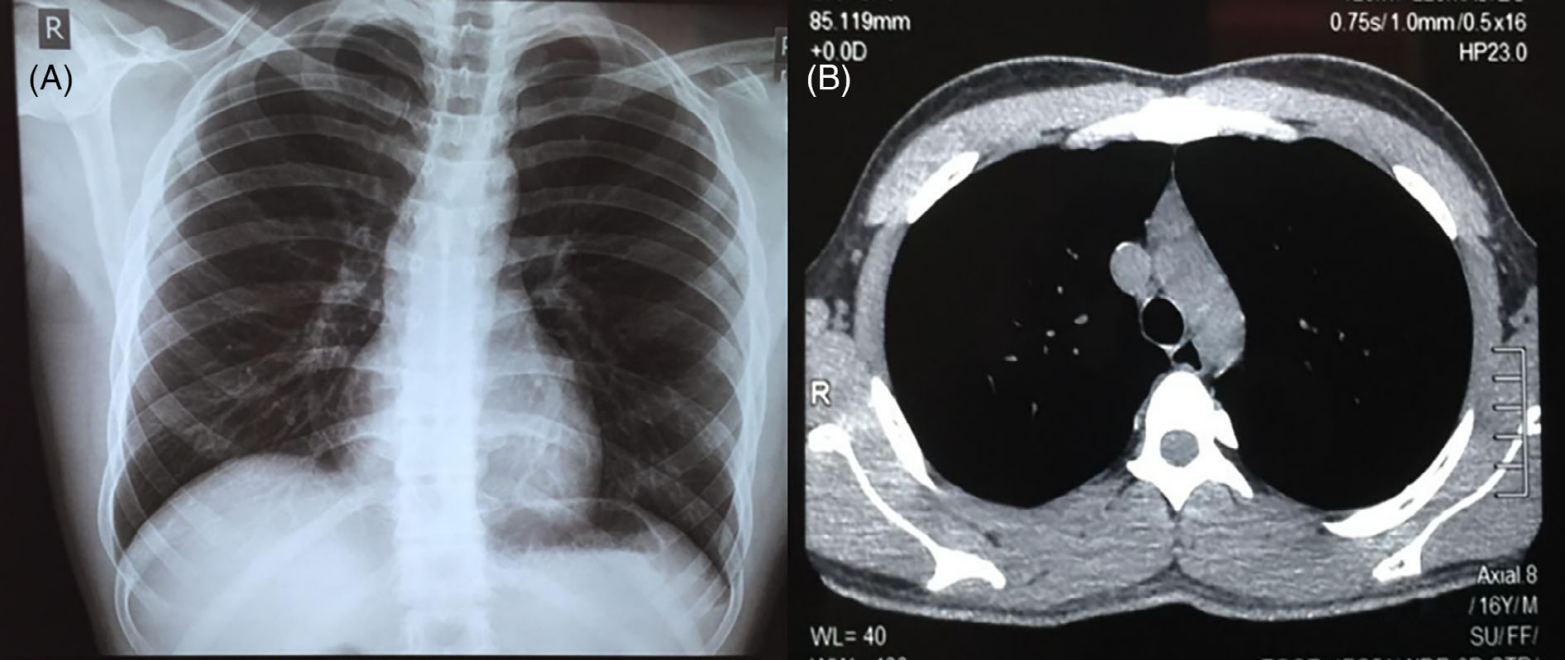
Chest imaging. (A) Chest radiography showing no radiological abnormalities both in the heart and lungs. (B) Computed tomography (CT) scan of the chest at that time showed no visible picture of acute lung inflammation, lung mass, or mediastinal tumour.
